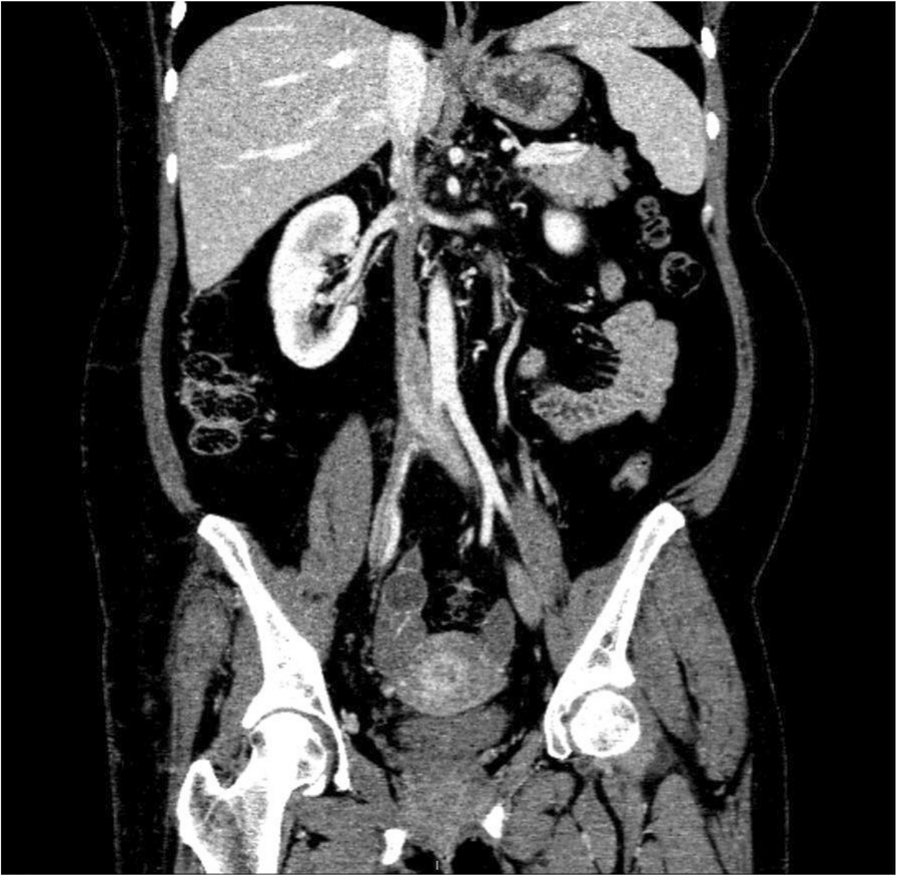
Computed tomography scan showing extensive thrombosis of the inferior vena cava.
